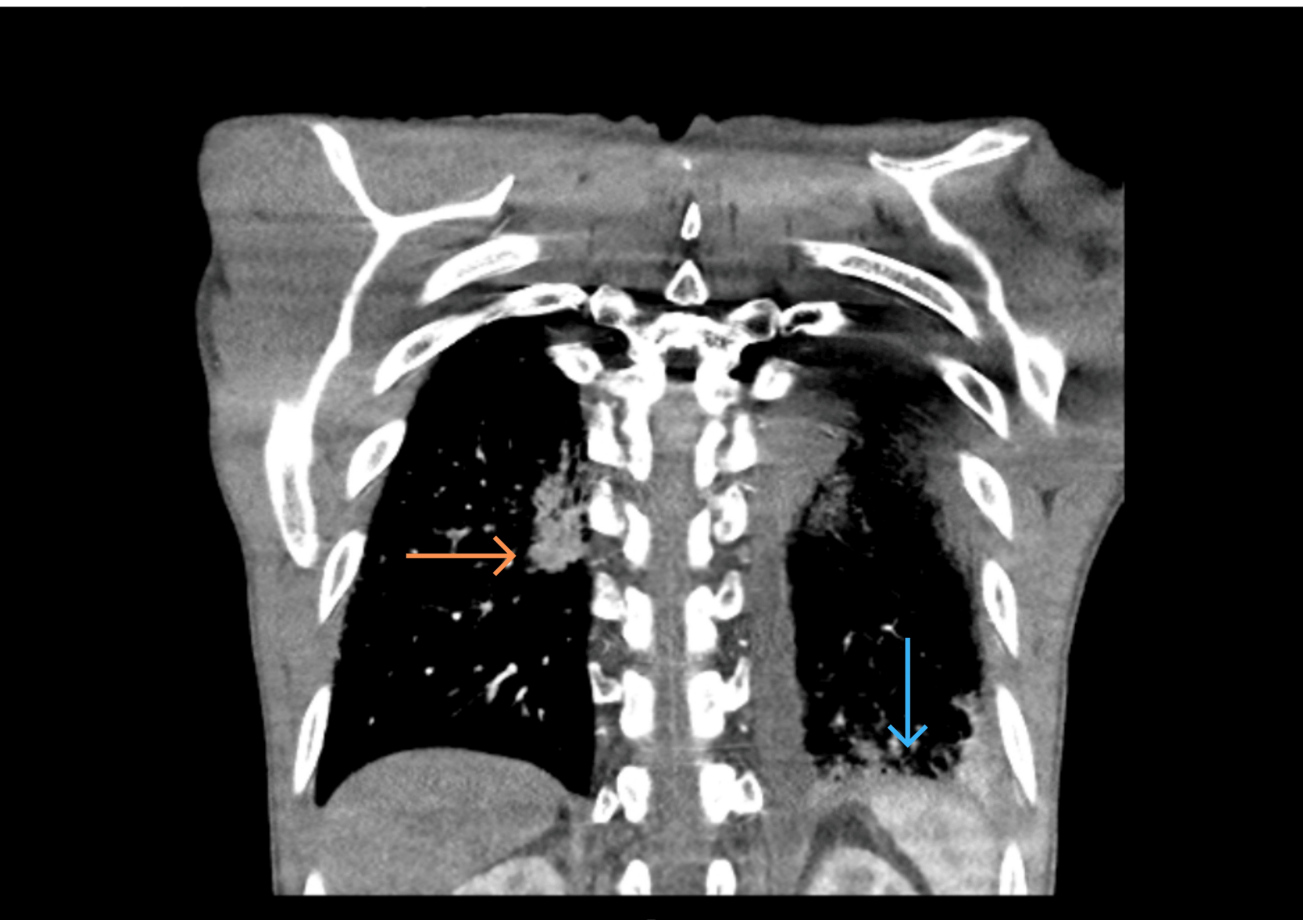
CTPA coronal view – right subpleural nodular consolidation (orange arrow) and consolidative changes in the left lower lobe (blue arrow) CTPA: Computed tomography pulmonary angiogram
